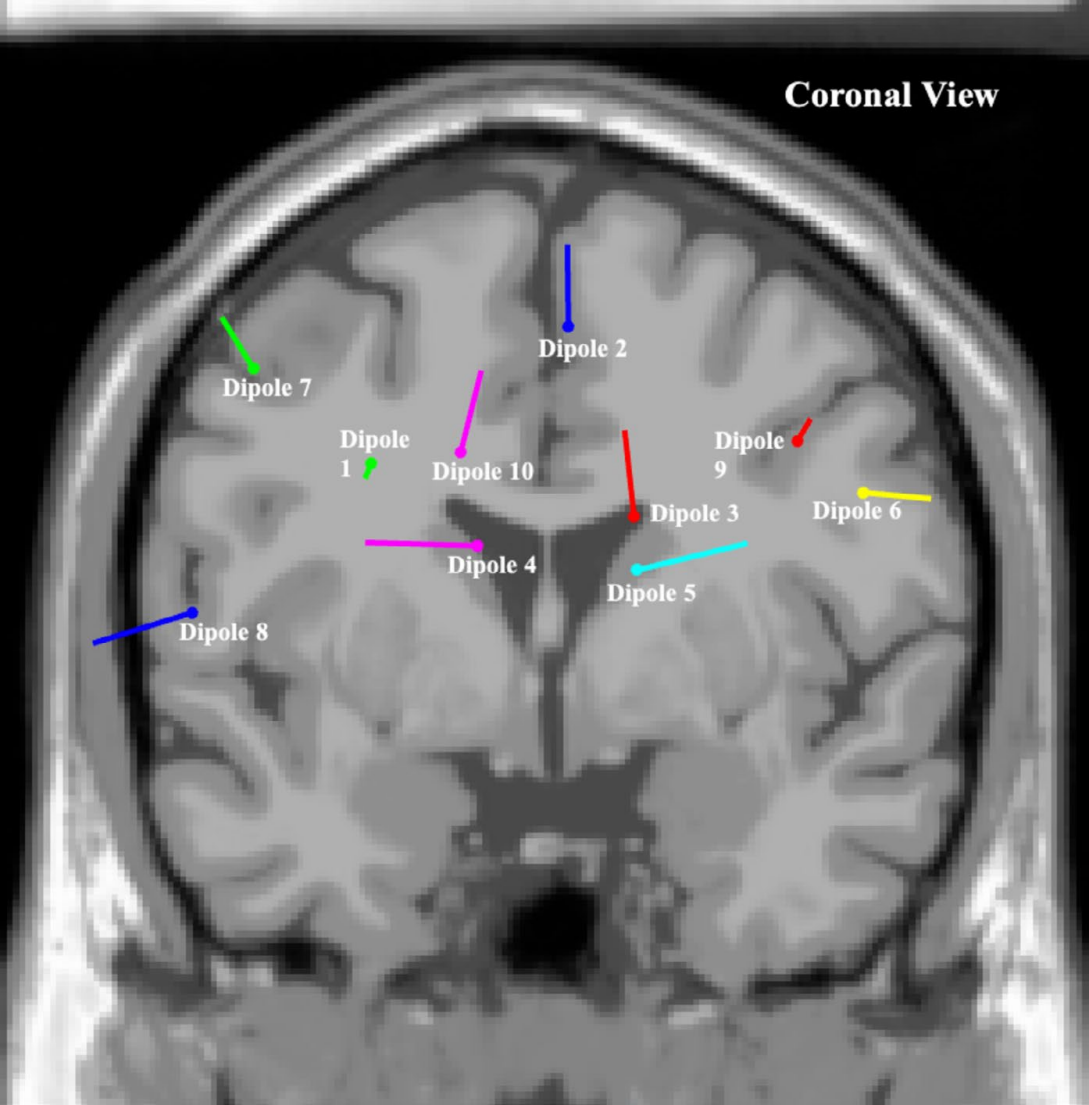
Coronal view of most significant dipole locations superimposed on an averaged referenced MRI.
